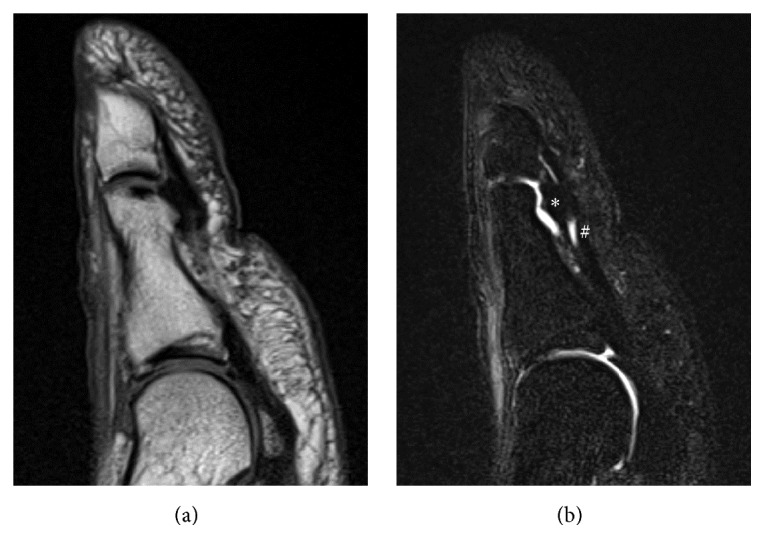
Sagittal T1 and T2 weighted magnetic resonance (MR) images showed a nodule in the plantar capsule at the interphalangeal joint of the great toe and fluid retention around it and the flexor hallucis longus tendon. (a) T1 weighted MR image. (b) T2 weighted MR image. ^*∗*^Plantar capsule nodule. ^#^Flexor hallucis longus.
